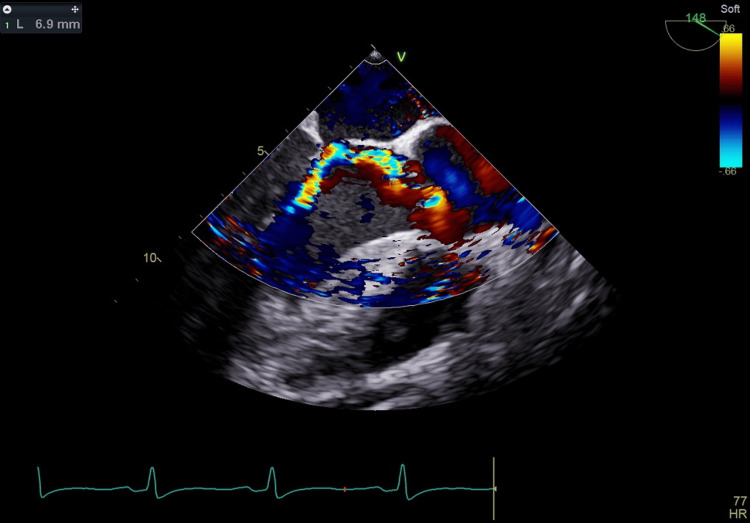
Severe AR assessed by the aortic valve long-axis view of TEE after induction of anesthesia A severe AR jet with an eccentric jet was observed from a point slightly to the right of the mid-aortic valve toward the anterior mitral valve wall. AR: aortic regurgitation; TEE: transesophageal echocardiography
